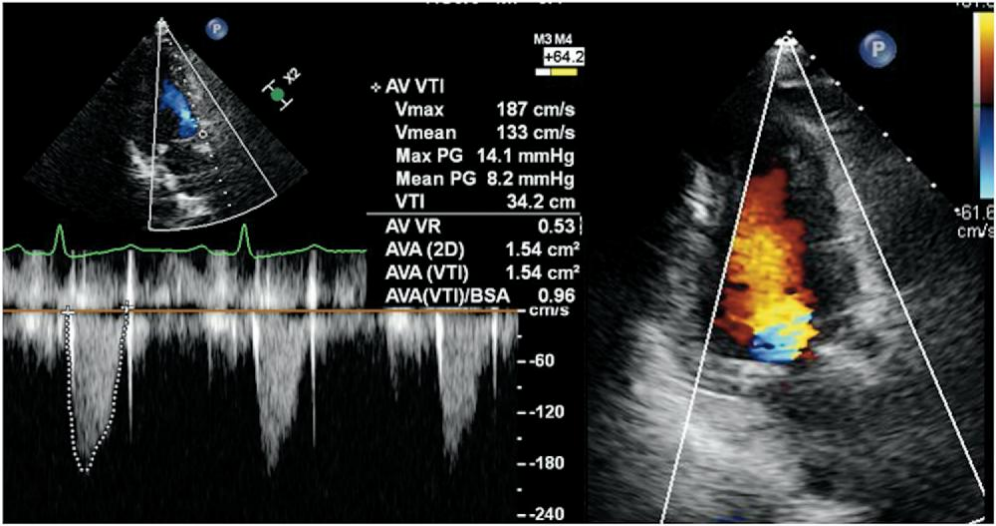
The post-operative transthoracic echocardiography demonstrated an improvement in pressure gradient, with no evidence of paravalvular leak.
